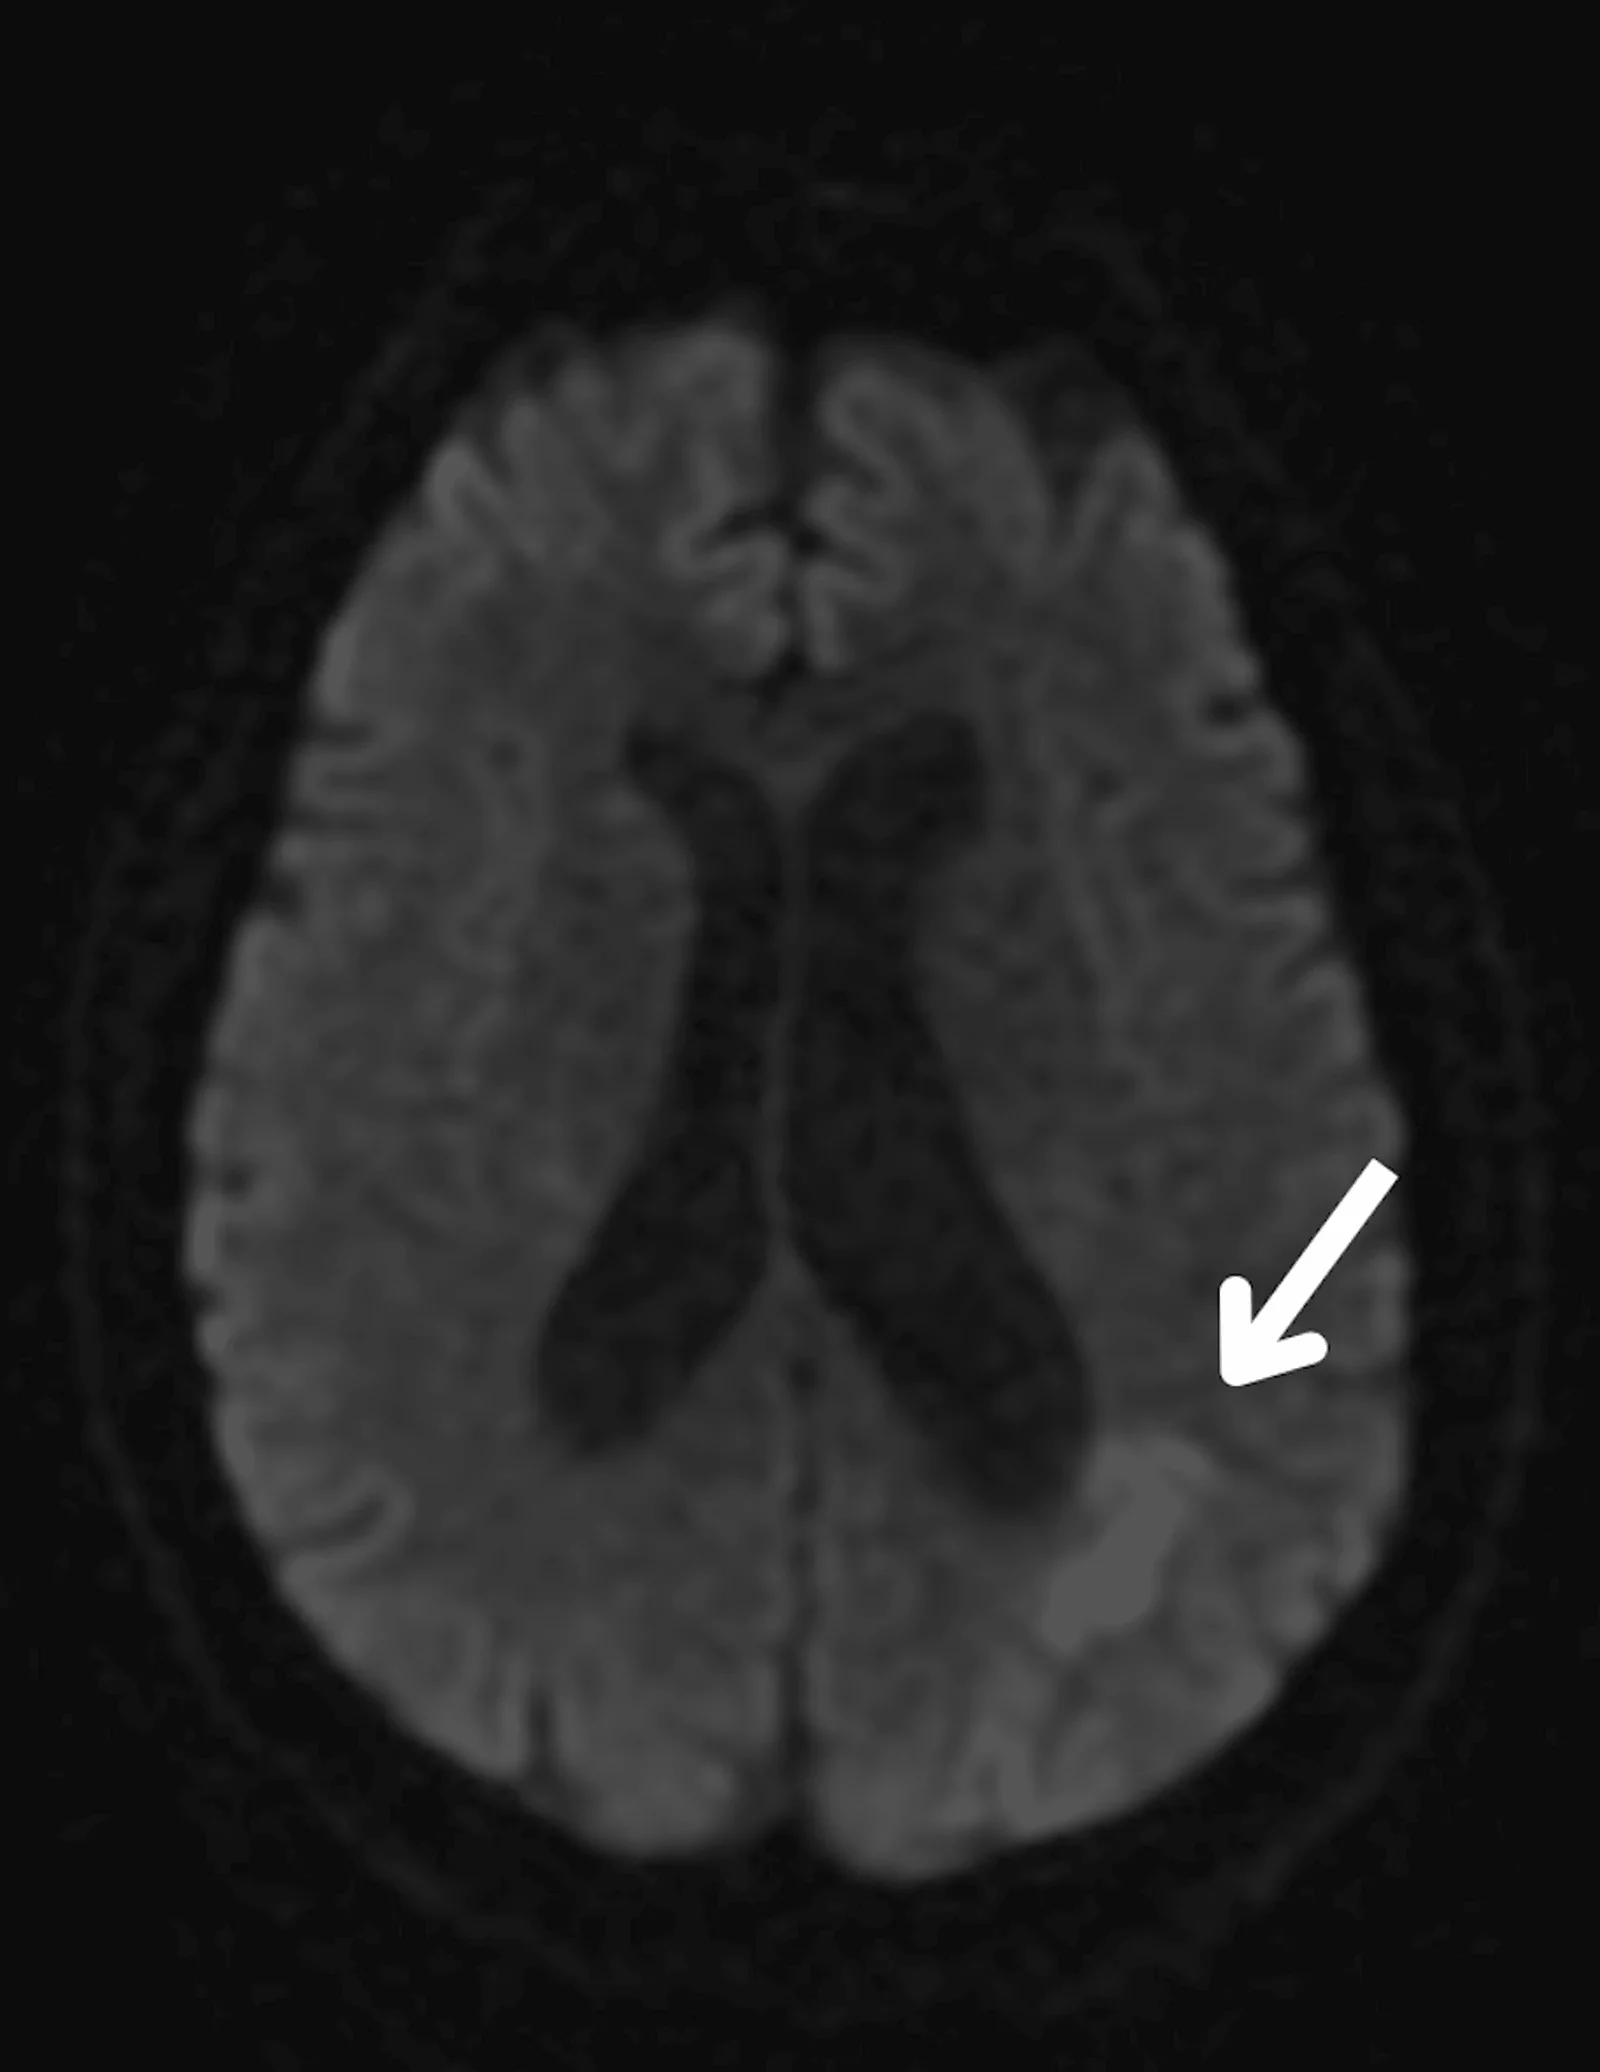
Magnetic resonance imaging (MRI) brain without contrast, diffusion-weighted imaging (DWI) sequence Arrow indicating an area of diffusion restriction in the left parieto-occipital white matter, consistent with an acute/subacute infarction in the setting of septic embolization
